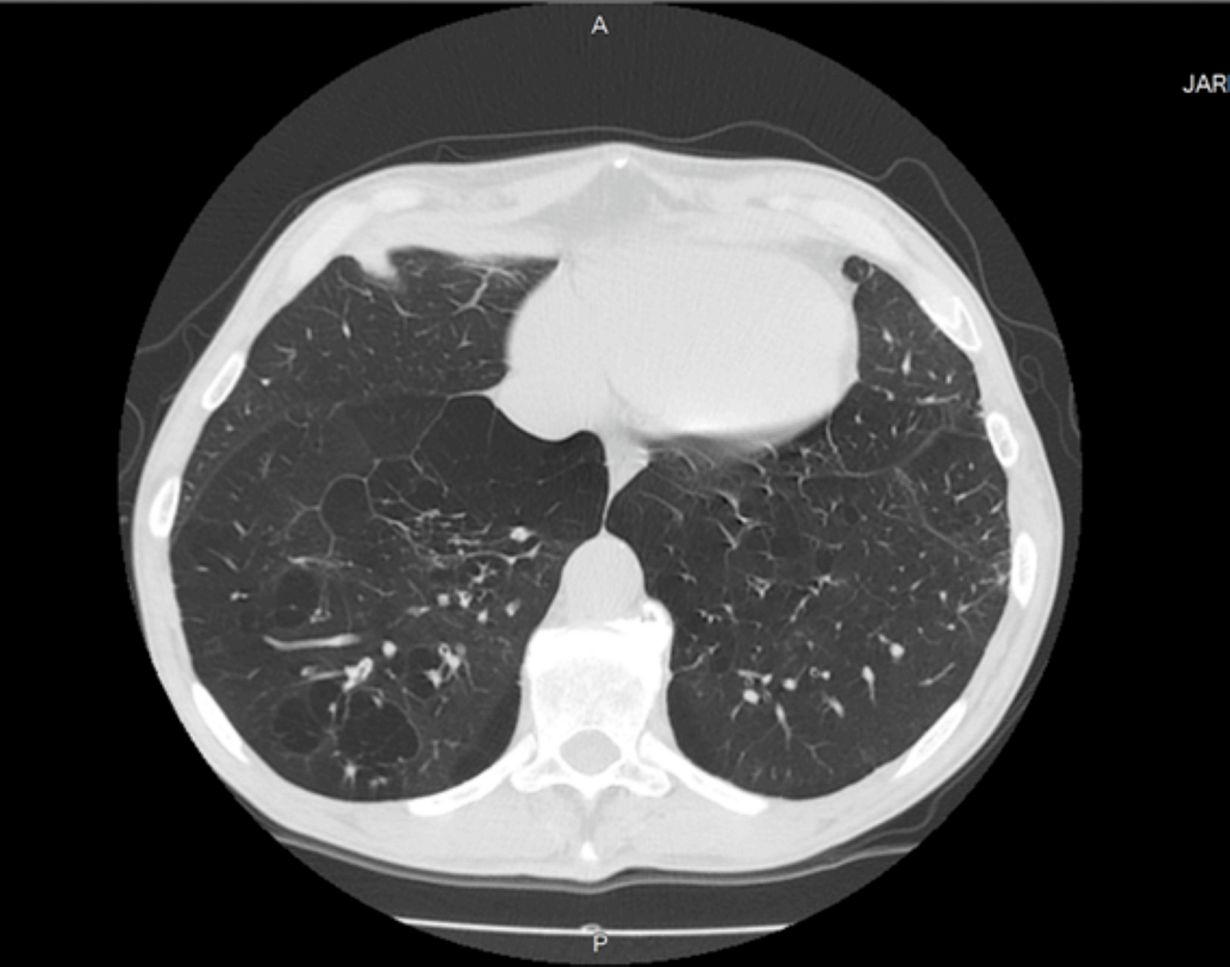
CT scan, at the lung basis' plane, November 2022 Centriacinar emphysema with dispersed and multiple blebs, with an exuberant superomedial, paraseptal, and cross-mediastinal major pleural bleb. Preserved airway caliber; discrete peribronchitis, with thickening of the inferior bronchi walls. Bronchiolectasis and small distal blebs outline trapped secretions, mostly in the right lung base; varicosed middle segmental bronchus. Preserved central airways, with dystrophic parietal calcifications. Pulmonary arteries with a diameter of 34 mm, suggesting pulmonary hypertension. Discrete mediastinal ganglia with nonspecific characteristics. No pleural or pericardial effusion.
